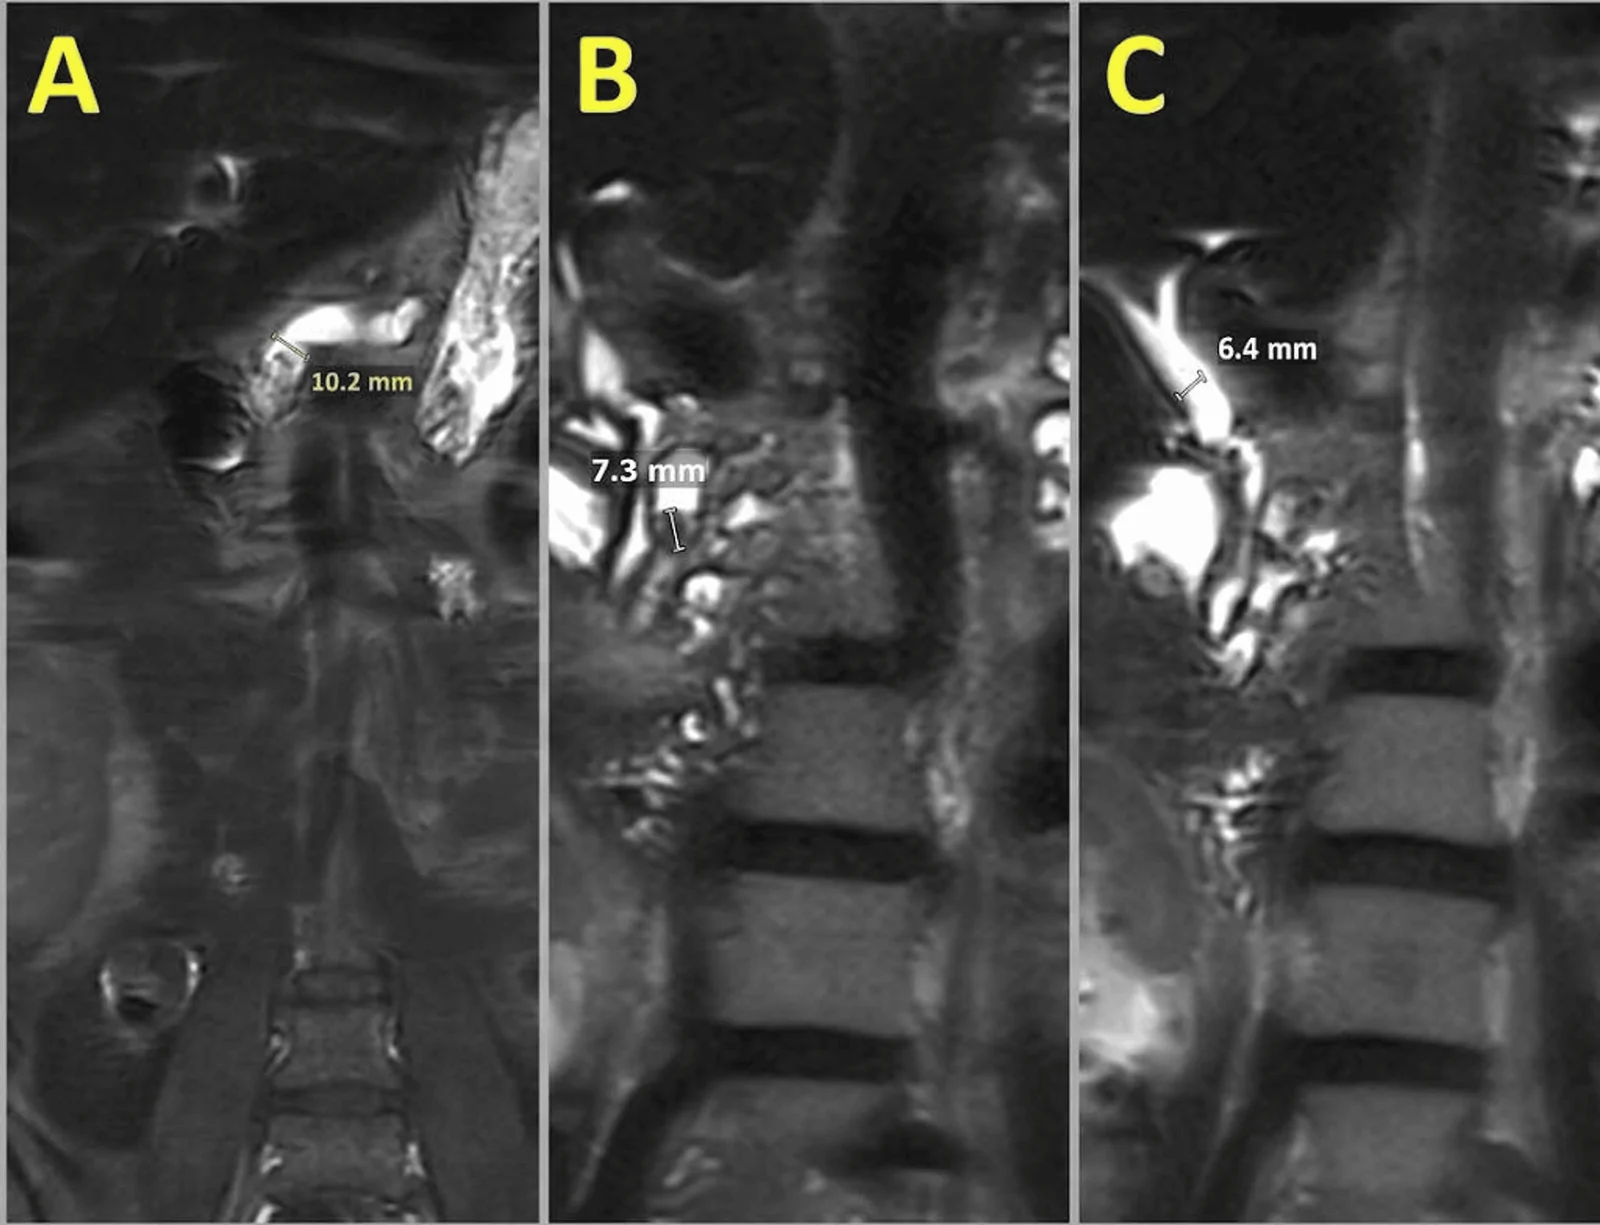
Magnetic resonance cholangiopancreatography (coronal T2-weighted). (A) Dilatation of the main pancreatic duct (MPD). (B) An MPD stone. (C) Dilatation of the common bile duct.
